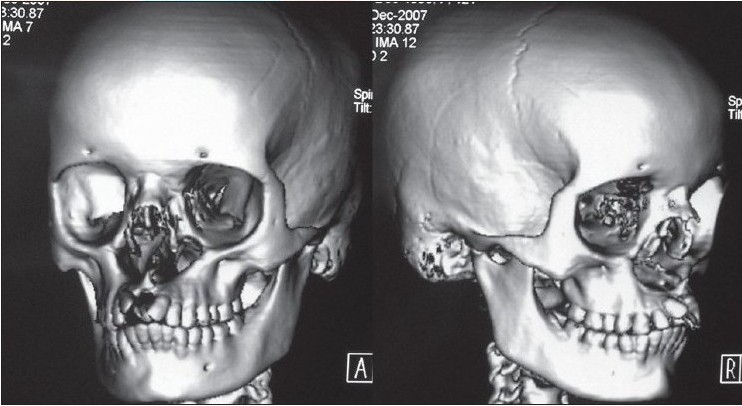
3-D CT scan of the same type-3 cleft patient showing severely hypoplastic nasal bone and maintained infra-orbital rim & orbital floor supporting the globe
